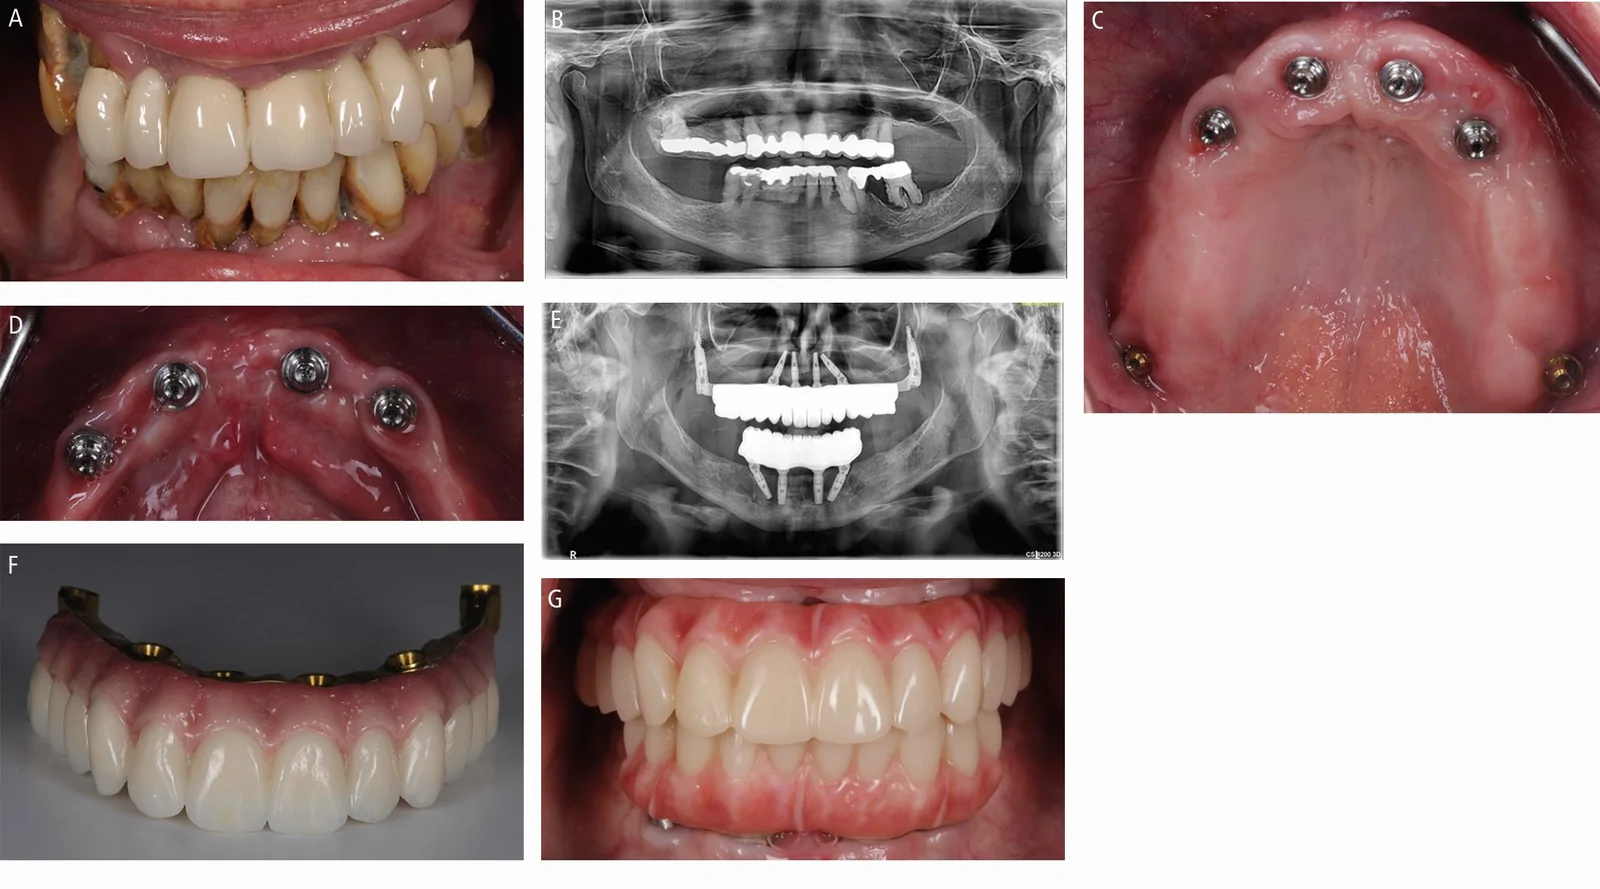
(A) Terminal dentition and bite collapse in a previously heavily restored dentition. (B) Pre-operative orthopantomogram (OPG) demonstrating advanced bone loss. (C) Upper arch treated with six implants. (D) Lower arch treated with four implants. (E) Post-operative OPG demonstrating appropriate spread of implants to allow for full arch fixed prosthesis. (F) Maxillary prosthesis. (G) Post-operative frontal view. Implants placed Dentsply Primetaper EV and Noris Pteryfit. Treating clinician Dr Ricky Bhopal (specialist prosthodontist) and laboratory work by Kevin Armstrong
